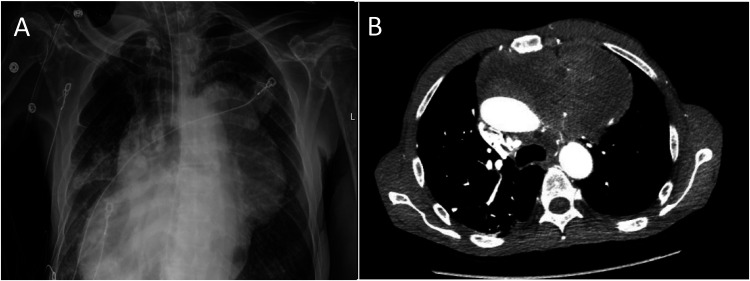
Chest X-ray demonstrating a large anterior superior mediastinal mass with displacement of the cardiac silhouette (A). CT scan showing the mass with extension to the left atrium, interatrial septum, lateral wall of the left ventricle, and left upper lobe of the lung (B).
